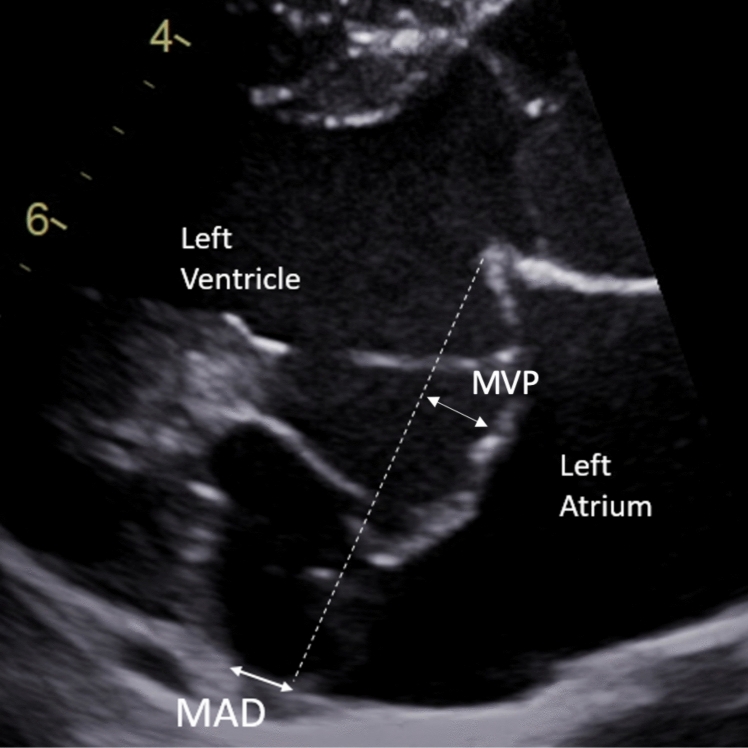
Parasternal long axis echocardiographic image showing atrial displacement of the posterior mitral valve leaflet hinge point within the AV junction (mitral annular disjunction) and mitral valve prolapse
